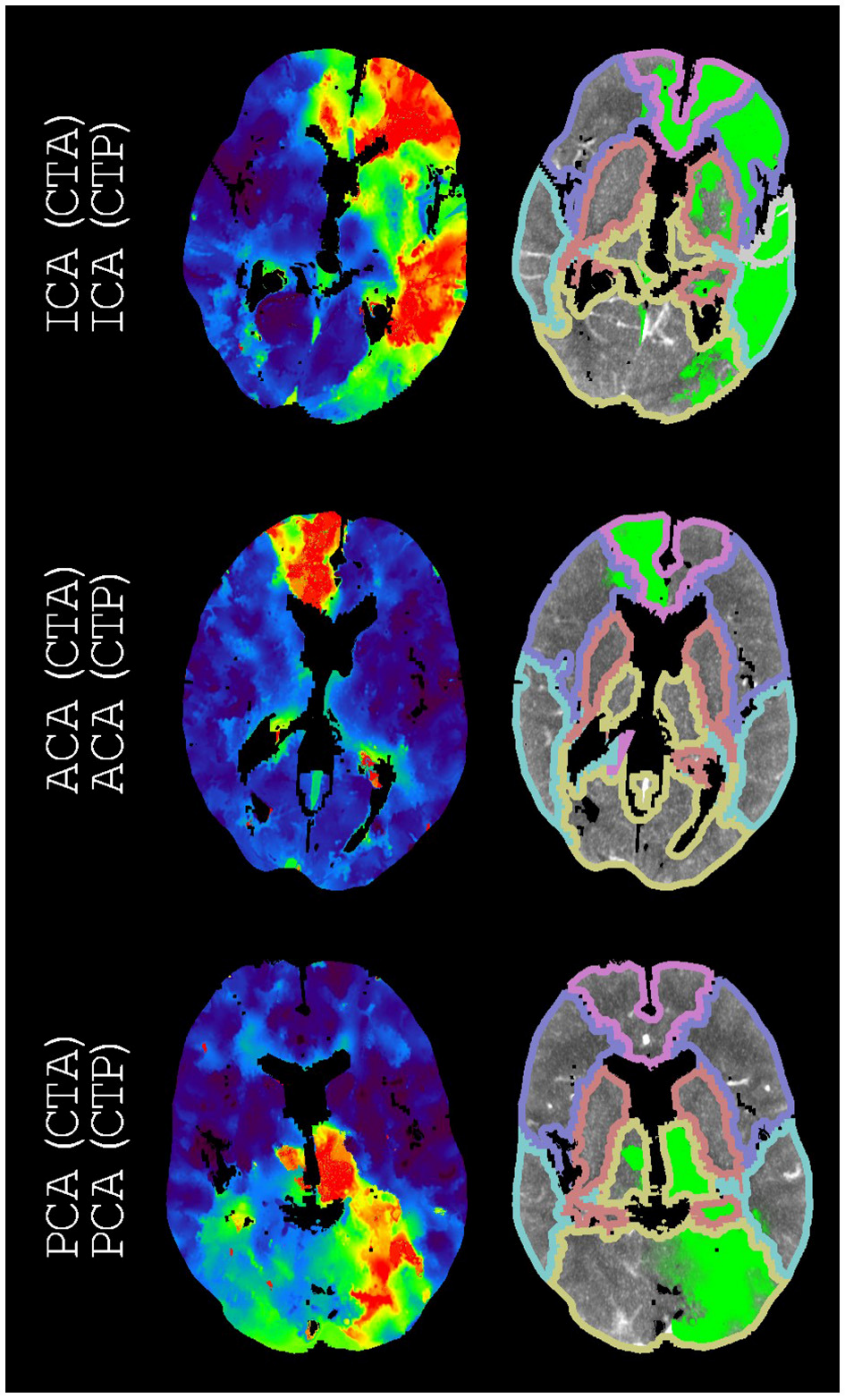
Examples of annotations (CTA) and indications (CTP) that match arteries other than the middle cerebral artery. On the left, time-to-peak perfusion maps are shown. On the right, the hypoperfused regions and the downstream regions (according to Figure 1) are shown. For each example, we only regarded the M3 downstream region with the highest intersection-over-union. The only displayed M3 downstream region is the superior temporal region.
